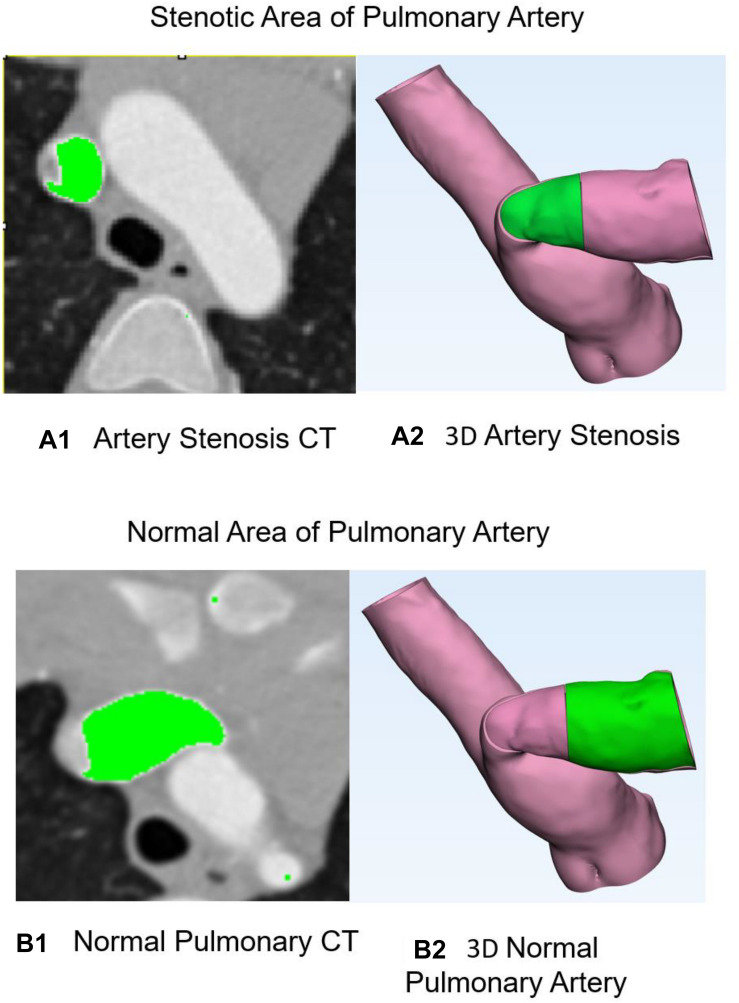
Stenosis and normal pulmonary artery. (A1) CT of pulmonary artery stenosis where the stenosis part of artery is marked in green. (A2) Three-dimensional artery with stenosis part in green. (B1) CT of pulmonary artery stenosis where the normal part of artery is marked in green. (B2) Three-dimensional artery with normal part in green.
